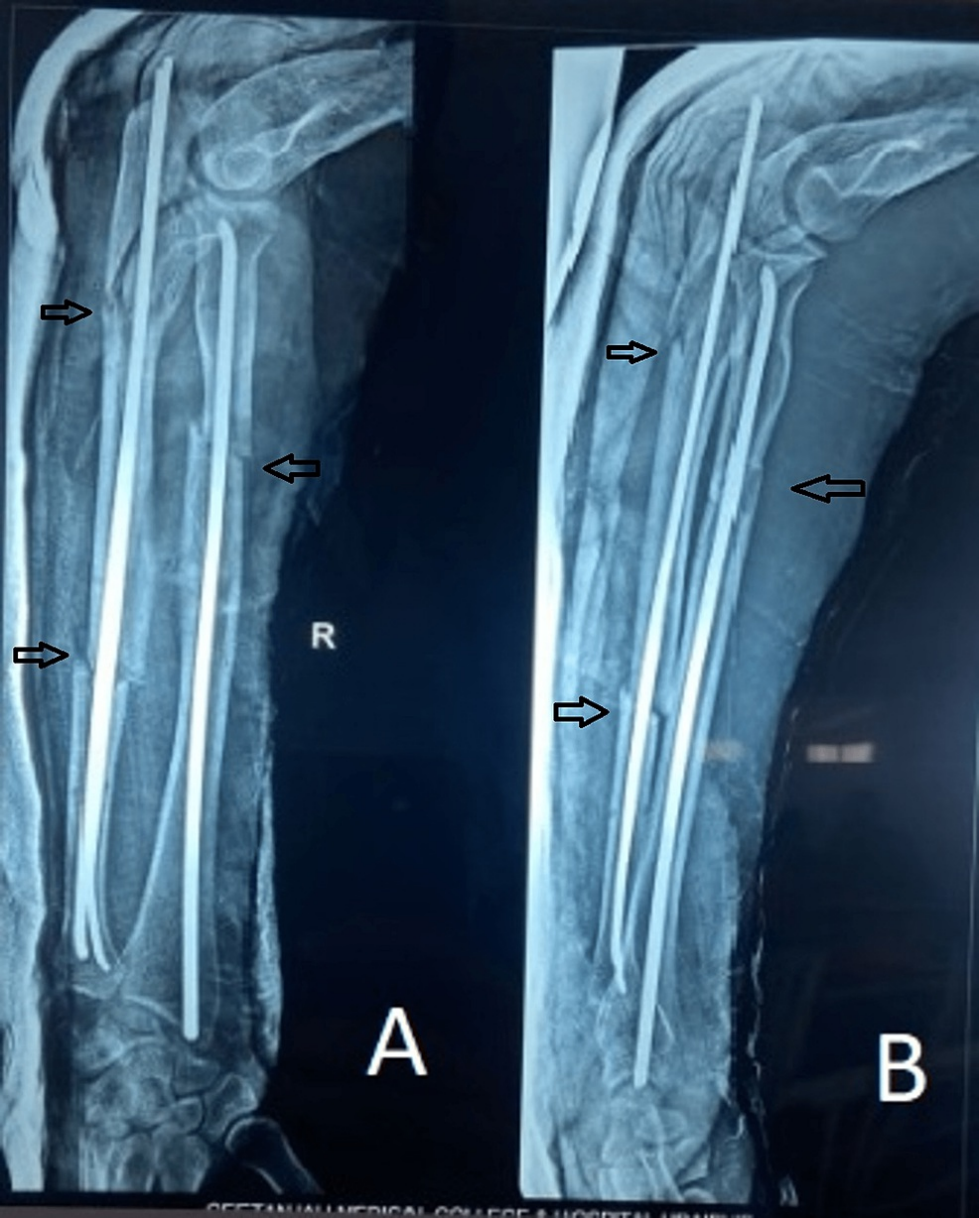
Post-operative radiograph. Post-operative radiograph showing fixation of the fracture (marked with arrows) with intramedullary titanium elastic nails. Figure A is the anteroposterior (AP) view, and figure B is the lateral view.
